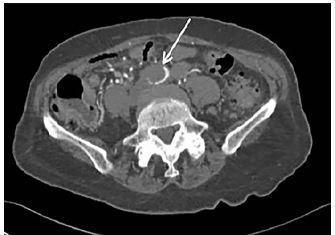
Computed tomography angiography axial image demonstrating occlusive thrombus (white arrow) in the distal abdominal aorta just proximal to the aortic bifurcation.
